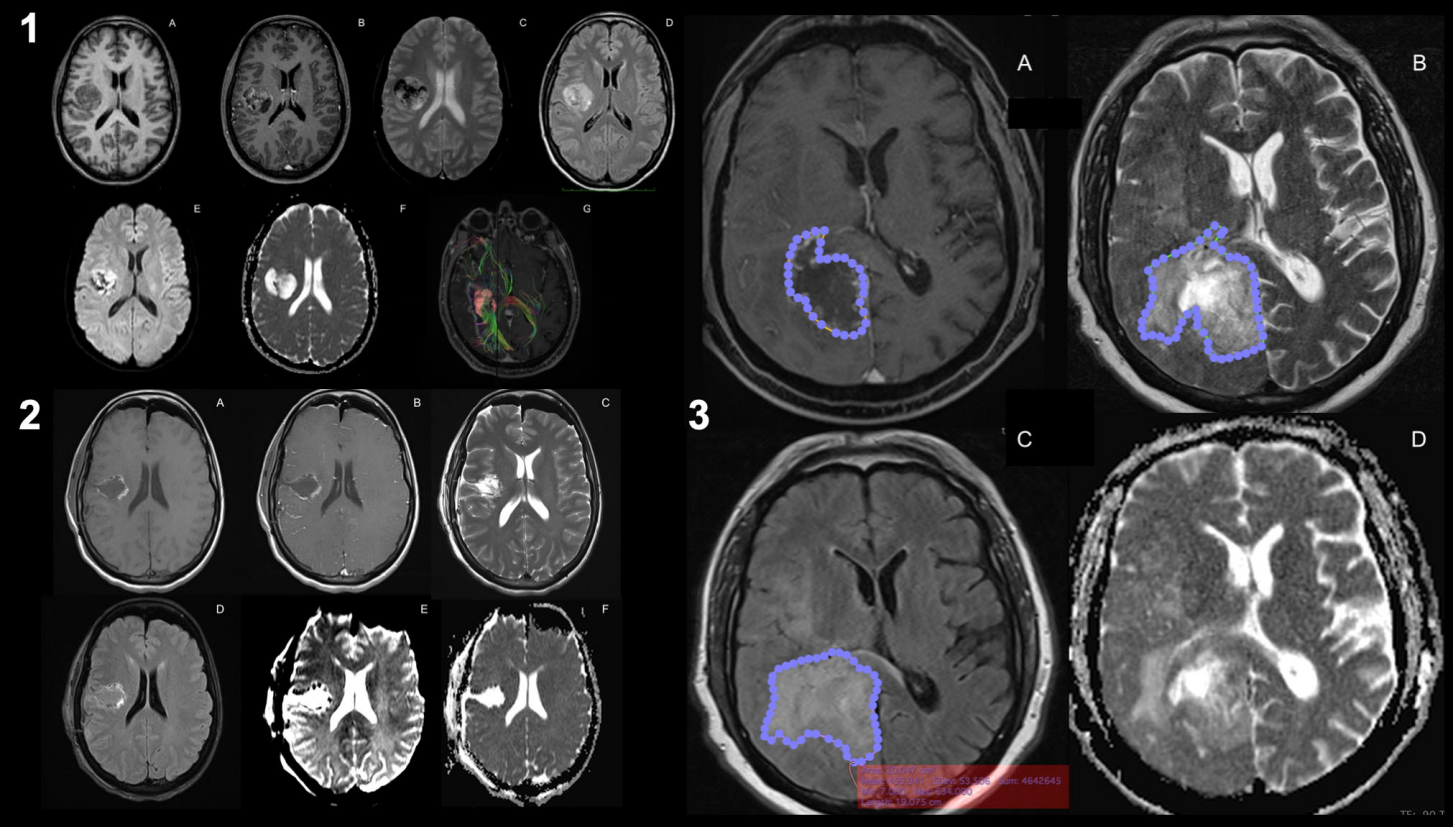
Magnetic resonance imaging of glioblastoma. Preoperative MRI-imaging sequences used for the study of glioblastoma (1): T1-weighted images (1.A), T1-weighted contrast-enhanced images (1.B), T2-weighted images (1.C), FLAIR images (1.D), perfusion imaging (PWI, 1.E), diffusion imaging (DWI, 1.F), and DTI with intra- and peri-lesional fiber-tracking study (1.G). Same sequences for the postoperative study of resection margins after the surgical procedure (2). Areas of the identified altered signals (3): the contrast-enhanced signal margin highlighted by a blue-dotted line (3.A), the signal margin in T2 associated with the presence of peri-lesional edema (3.B), the margin in the same lesion in FLAIR (3.C) and the DWI (3.D). It can be seen that there is a discrepancy between the signal area in FLAIR versus T2 (known as T2-FLAIR mismatch), which may imply that there are areas of PBZ within healthy edematous tissue.
